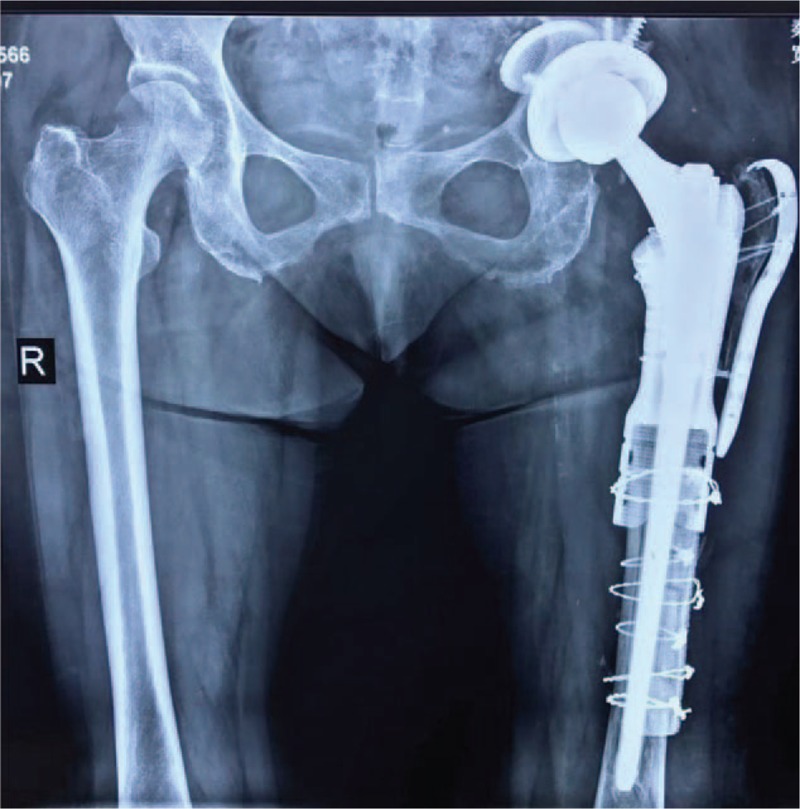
Postoperative radiograph demonstrated good restoration of hip center and reconstruction of proximal femur after surgery.
